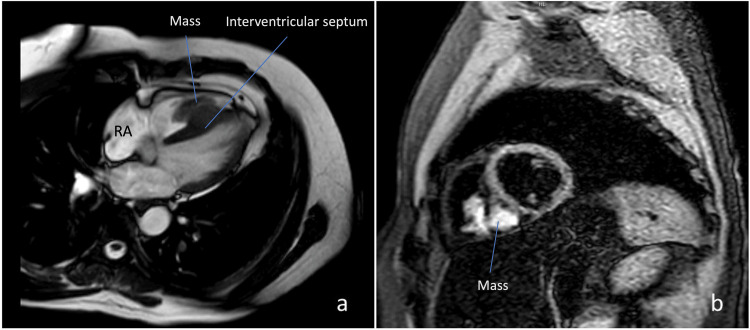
Magnetic resonance imaging of the mass attached to the interventricular septum (PSIR and sBTFE sequences). RA: right atrial; PSIR: phase-sensitive inversion recovery; sBTFE: saturation recovery with balanced turbo field echo pulse.
